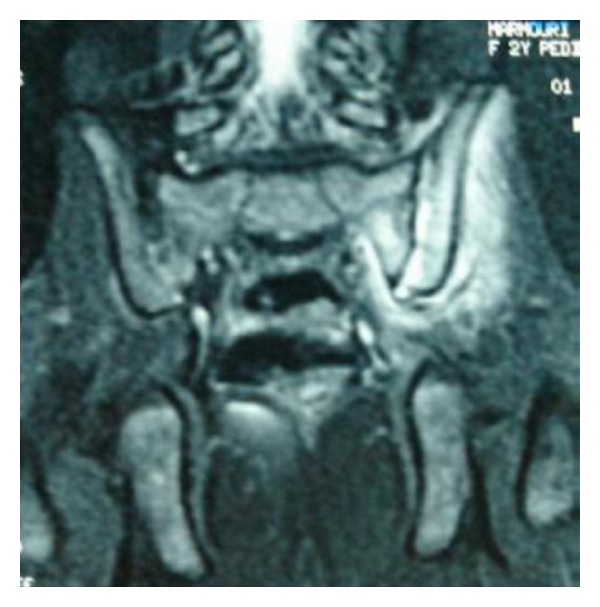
MRI of the pelvis: on T2-weighted images an increase of the signal intensities of the left sacroiliac joint and increase of the signal of the iliacus and gluteal muscles. Also there was a little effusion in the left sacroiliac joint.
